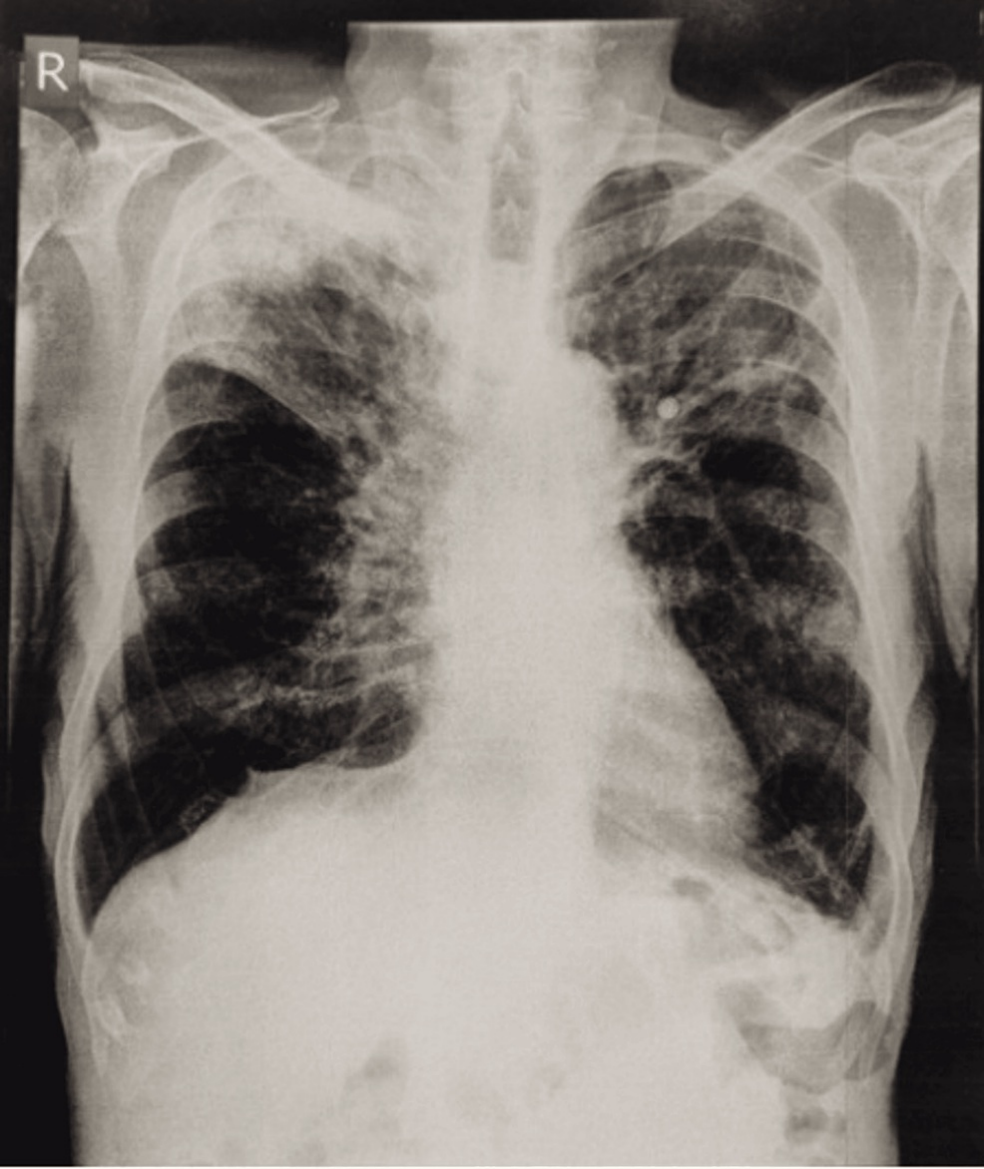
Chest radiograph showed bilateral upper zone and right middle zone inhomogeneous opacities with hyperinflated lung fields
